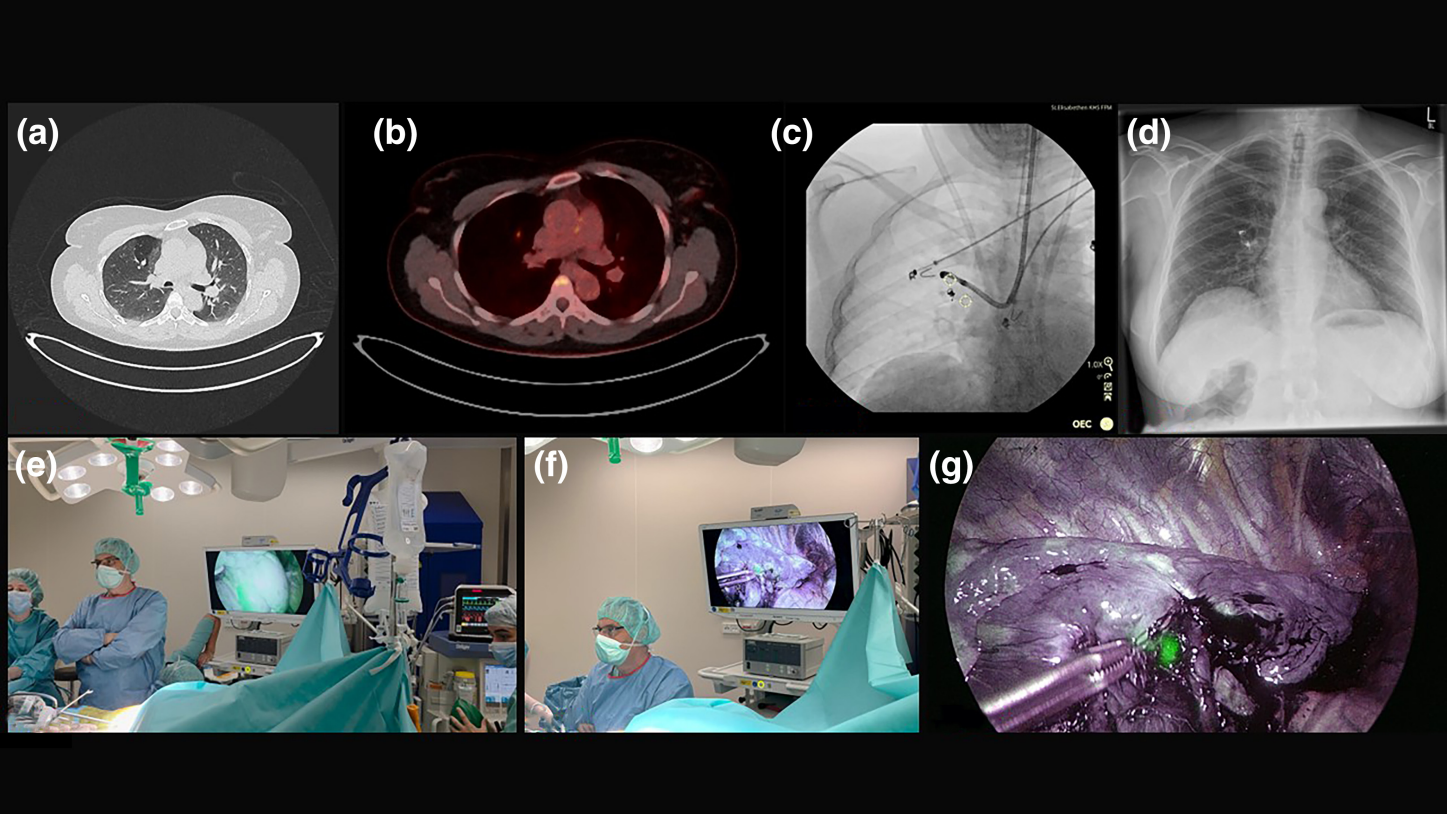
(a) CT scan showing lesion in right upper lobe (S3). (b) PET‐CT scan with FDG uptake in the right upper lobe lesion. (c) Dual coil‐placement in right upper lobe under fluoroscopic guidance. (d) Postinterventional chest x‐ray showing positioning of both coils in right upper lobe. (e) NIR thoracoscopy identification of first coil adjacent to the pleura. (f and g) NIR thoracoscopy identification of second coil adjacent to the tumour.
